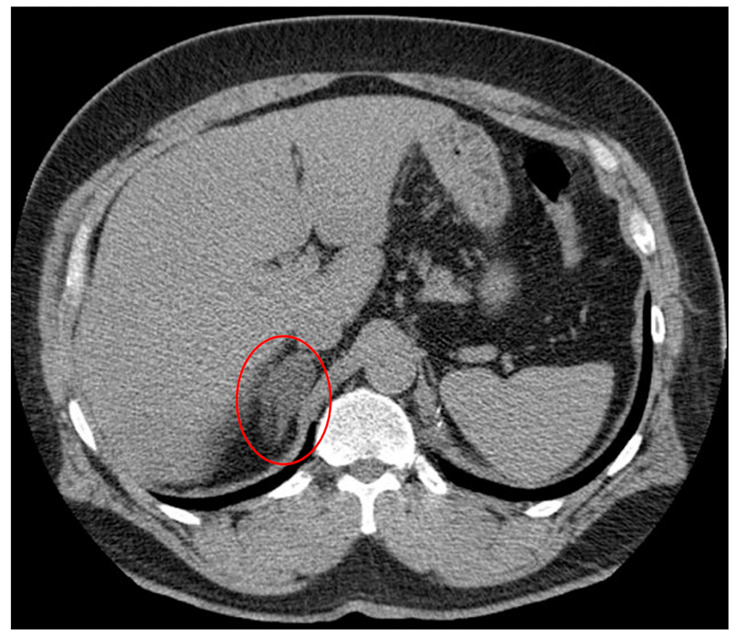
Incidental right adrenal nodule discovered on non-contrast CT. Case courtesy of Dr. Hani Makky Al Salam, radiopaedia.org. rID:10109 [9].
